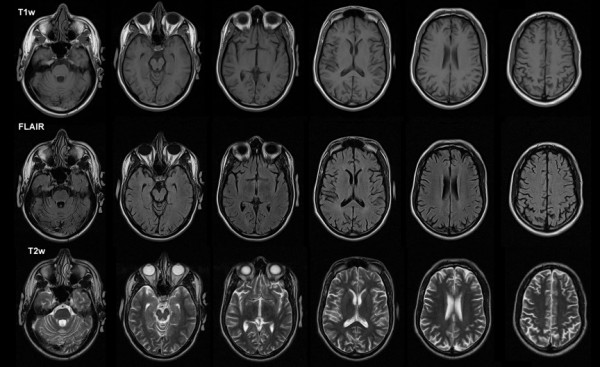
Representative axial slices from clinical MRI scans of the TBI patient. T1-weighted (top row), FLAIR (middle row) and T2-weighted images. The scans were interpreted as normal at the initial neuroradiological examination. Images are displayed according to radiological convention (right side of the brain is shown on the left side of figure).
